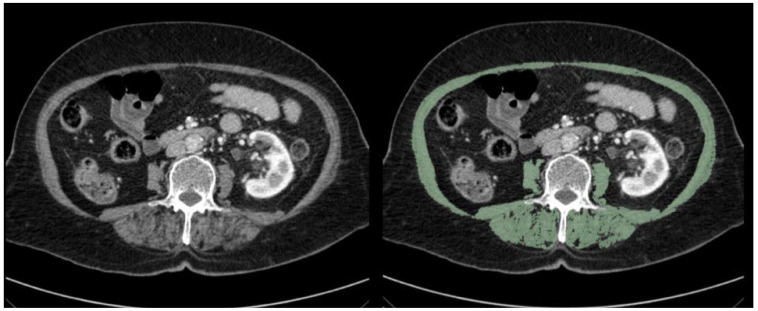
CT L3 Vertebra Level Skeletal Muscle Area Calculation: In the CT image on the left, the L3 vertebra level before scanning is shown using the 3D-Slicer application, while the green-shaded areas in the CT image on the right represent the skeletal muscle areas scanned with 3D-Slicer at the same level.
